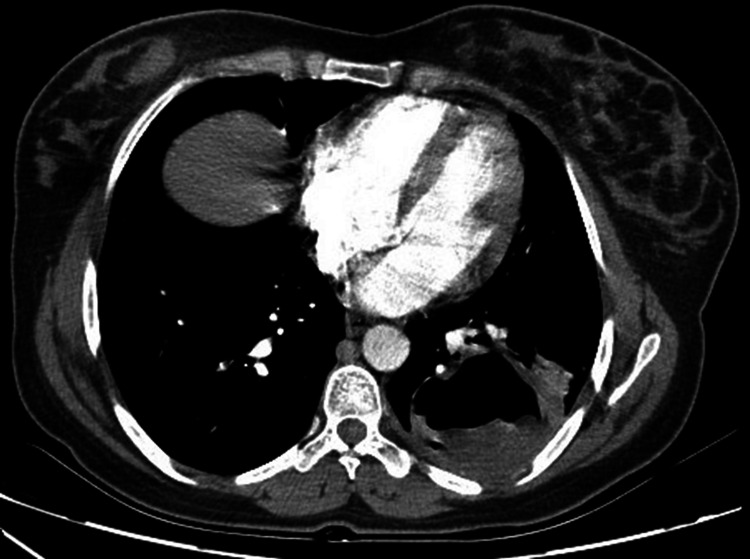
Axial chest CT image in lung window showing a cavitary lesion of the left lower lobe communicating with the bronchial tree, associated with perilesional ground-glass opacities consistent with alveolar hemorrhage, suggestive of a ruptured pulmonary hydatid cyst.
